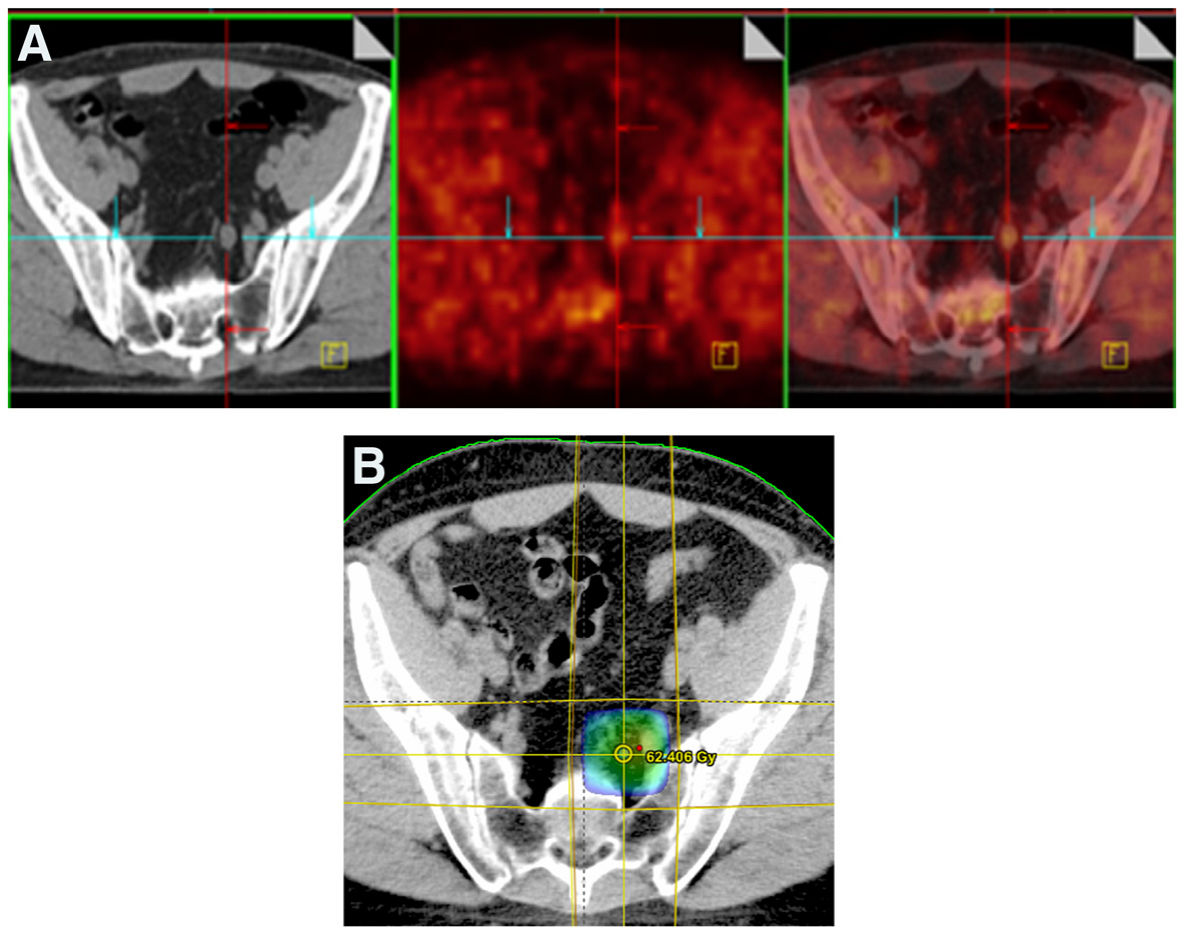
(A)^18^ F-FCH PET/CT (patient no. 3) showing a transverse section with a suspicious lymph node metastasis in the left internal iliac region. (B) The corresponding color wash dose distributions for the planning target volume (PTV).
